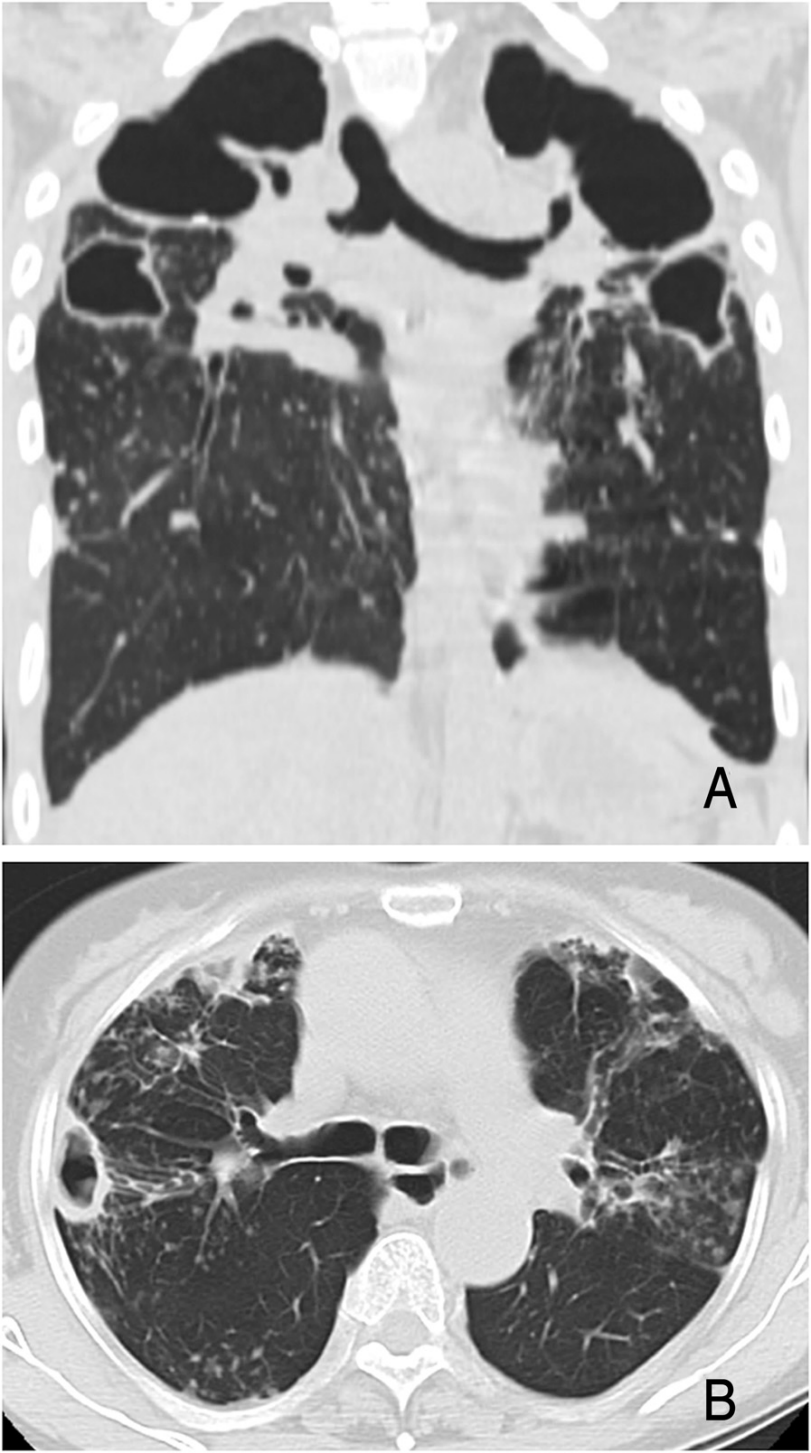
High-resolution computed tomography (HRCT) findings of “*M. indicus pranii*” lung disease. a A 27-year-old male with a prior history of pulmonary tuberculosis. Chest HRCT shows multiple bilateral large cavities in both upper lobes. The patient died after initiation of combination antibiotic therapy due to an accident. b A 72-year-old female. Chest HRCT shows severe bronchiectasis in the right middle lobe and lingular segment of the left upper lobe. Note a cavitary lesion in the right middle lobe, as well as multiple small nodules and tree-in-bud appearance in both lungs
